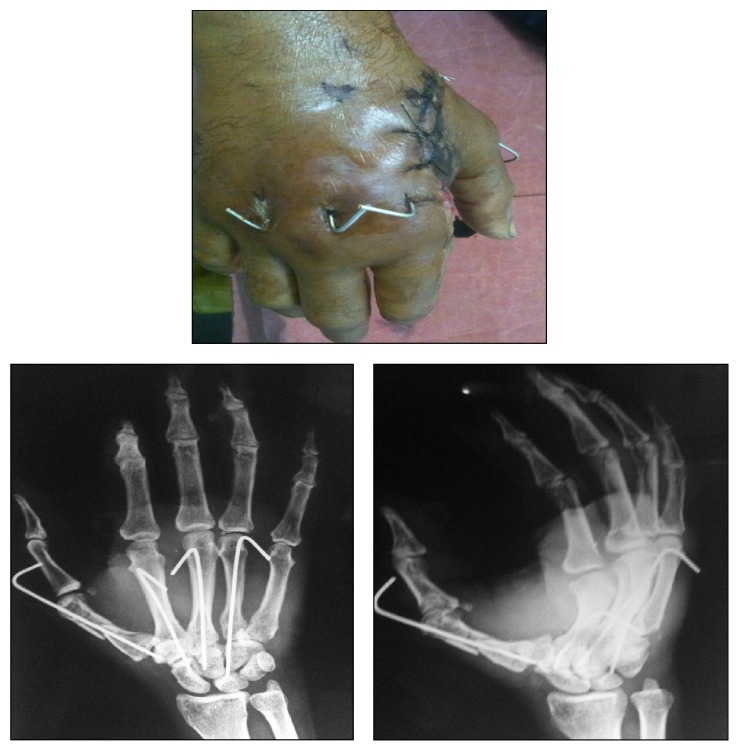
Clinical picture and X-ray at 2 weeks.
